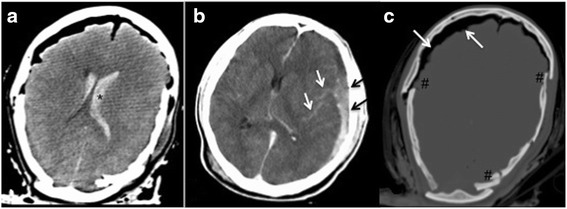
The soft-tissue-window of a cerebral CT of a young patient demonstrates the advantages of pmCT in terms of clearly showing intraventricular hemorrhage (a,*), subarachnoidal bleeding (see *white arrows*) along with subdural hematoma (see *black arrows*) on the left side (see (b) without changing the pressure-in situ. The display of CT in the bone window additionally demonstrates the multiple fragment fracture (#) of the calvarium as well as massive free intracranial air (see *white arrows*) (c)
